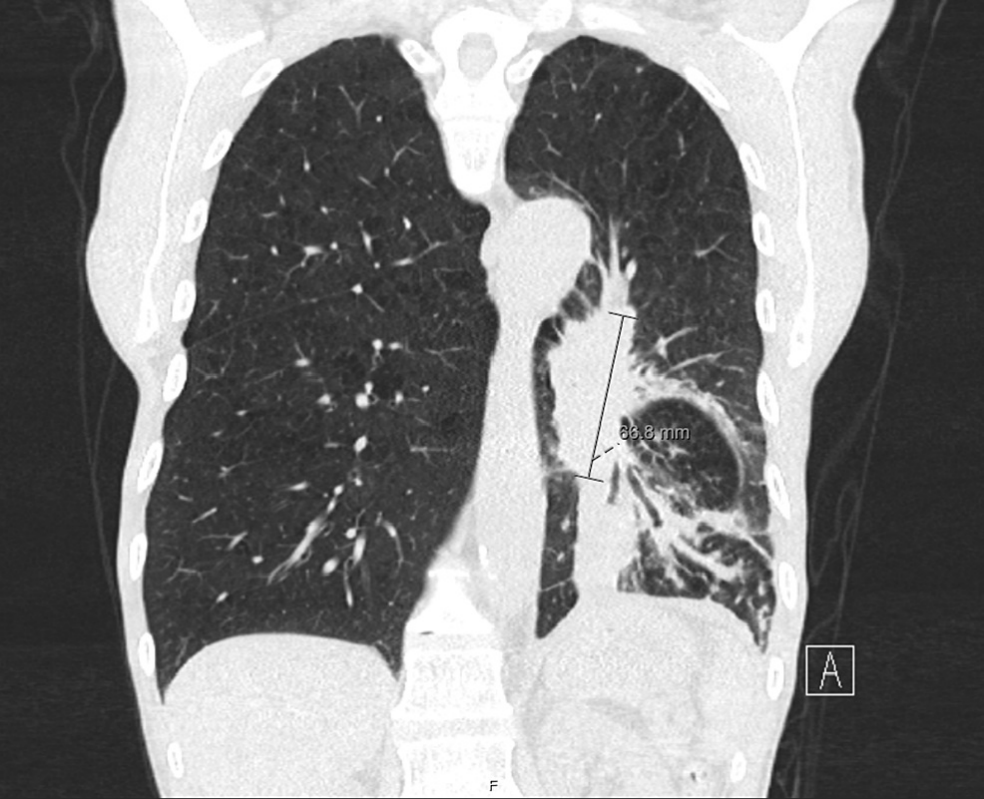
Lung peri-hilar mass hiding behind the cardiac shadow In the coronal view, we can see that the lung mass, albeit its important size, is largely hidden behind the cardiac silhouette, which justified why it was not apparent in the posteroanterior view of the X-ray.
